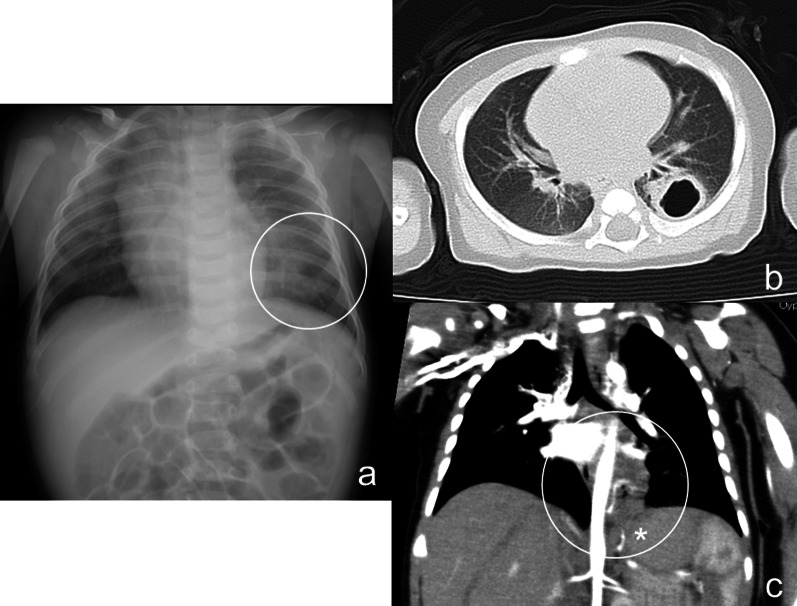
The chest radiograph showed a ring-shaped infiltration 2 cm in diameter in the lower left lung field (a). Contrast-enhanced computed tomography (b, c). CT scans led to the diagnosis of a lung abscess in the left lower intralobar pulmonary sequestration forming a tumor-like lesion with aberrant arteries (asterisk) from the abdominal cavity
